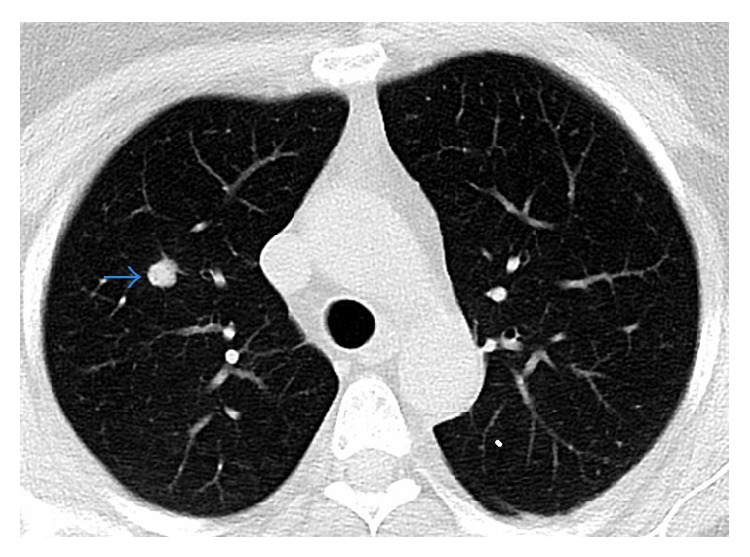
Thorax CT showing lesion in the right lung.
